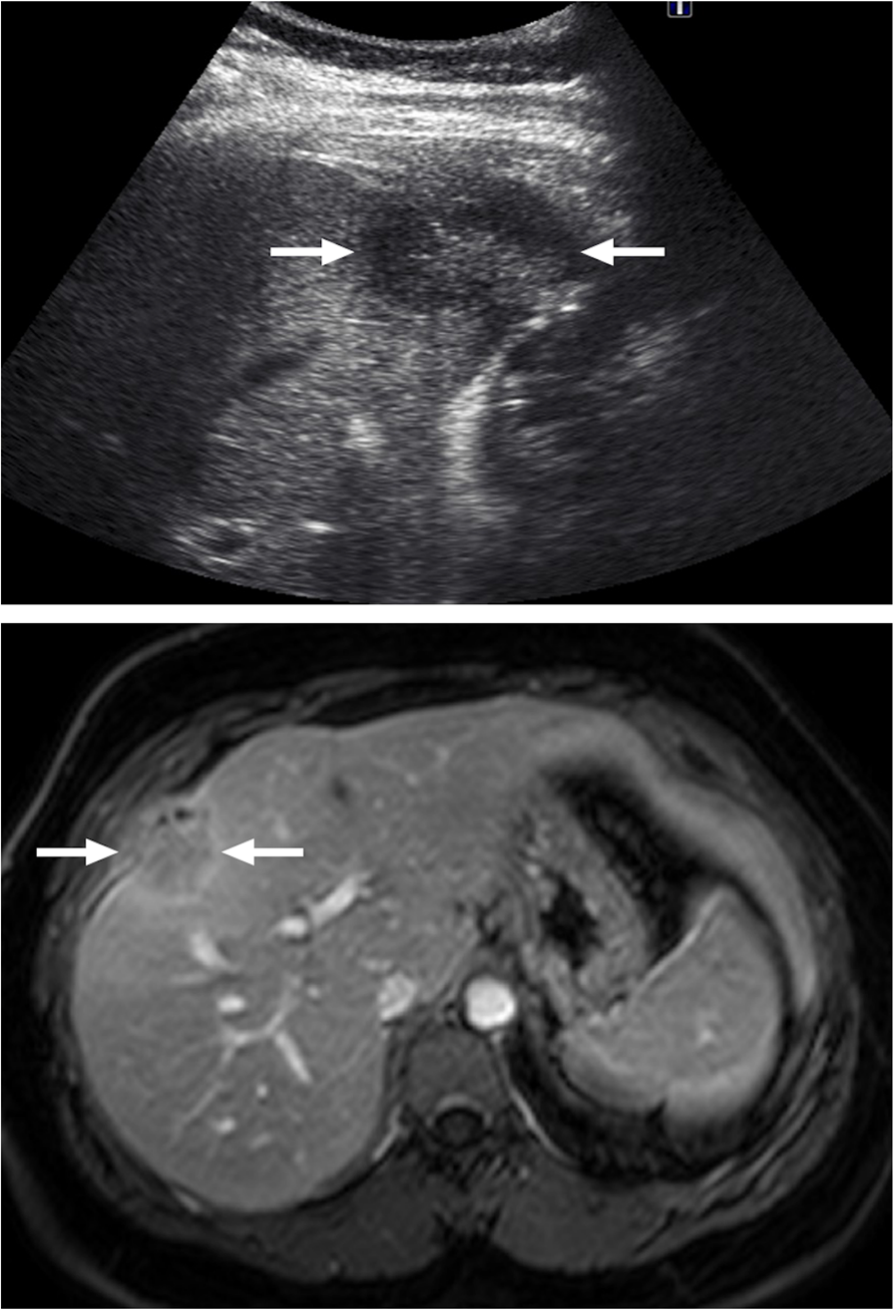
A 37-year-old female with no known systemic disease presented with right upper quadrant pain. a Abdominal ultrasound demonstrates hypoechoic solid mass lesion with slightly increased central echogenicity (arrows). b Axial plane postcontrast T1W image demonstrates peripherally enhancing infiltrative sub-capsular mass lesion (arrows) extending into perihepatic area and infiltrating abdominal wall fat. Also note associated peritoneal thickening. Percutaneous US-guided biopsy revealed granulomatous inflammation consistent with tuberculoma
